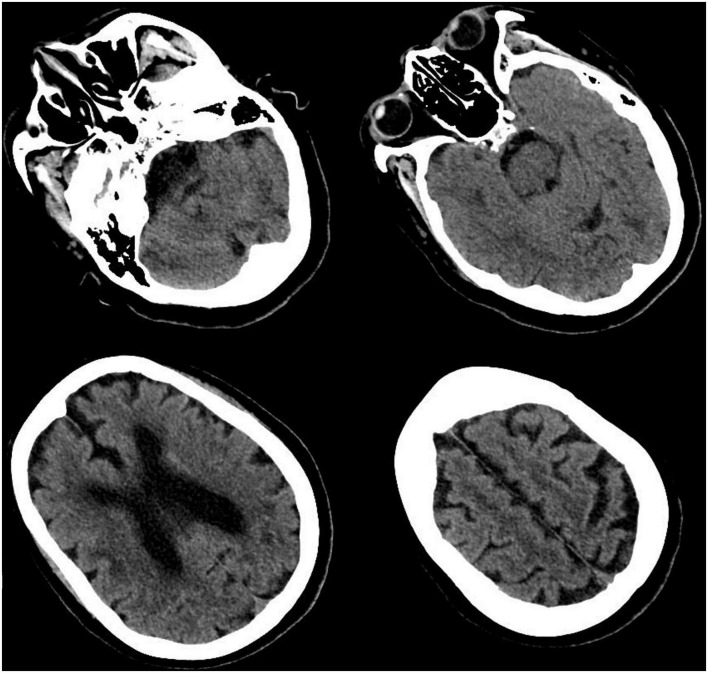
Cranial computed tomography (CT) scan. CT scan showed multiple cerebral infarction, white matter degeneration, brain atrophy, with no sign of cerebral hemorrhage or space-occupying lesion.
